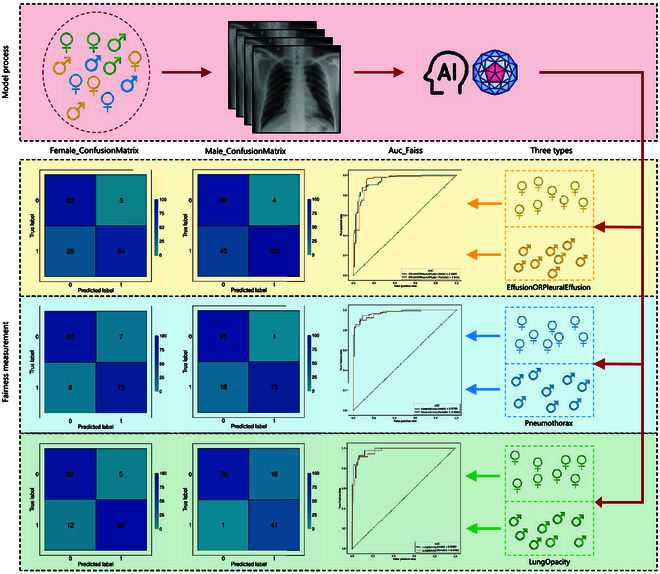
Fairness analysis of the PanCV model. Chest radiographs with diagnoses of “Effusion OR Pleural Effusion,” “Pneumothorax,” and “Lung Opacity” were used to verify the fairness of the AI model; specifically, we tested whether the model’s classification differed between male and female chest radiographs. The above 3 labels represent the 3 categories of the model's worst, second-worst, and median (AUC) performance on the validation set.
